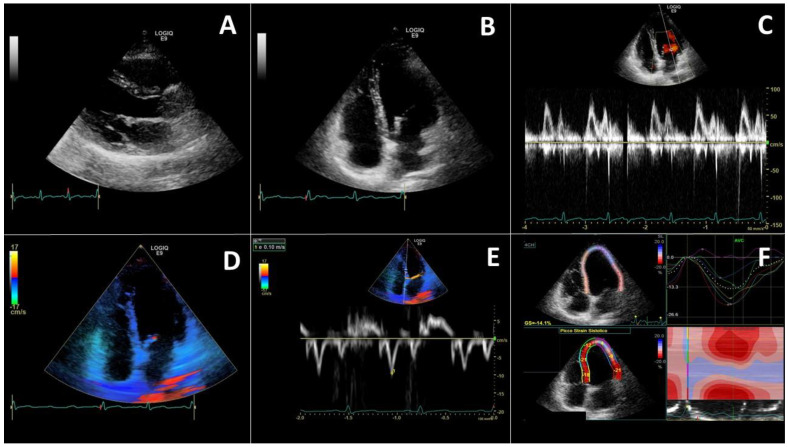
Left heart evaluation needs a complete echocardiography evaluating parasternal long-axis (A) and apical 4 chamber (B) views. Using pulsed wave Doppler trans-mitral flow is evaluated (C). The apical 4 chamber view allows to perform tissue Doppler imaging analysis (D), able to make a doppler spectral analysis of the myocardial contraction velocity wave (E). A complete evaluation also includes the Speckle tracking evaluation to obtain the longitudinal strain (F). In particular the shown image was obtained in a 28-year old girl with a COVID-19 myocarditis.
